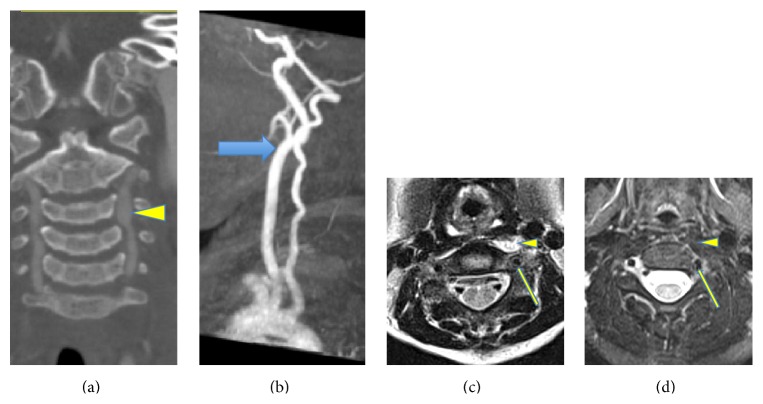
Neck imaging before and after treatment. (a) CTA coronal reformats demonstrating bilateral vertebral arteries prior to treatment. There is focal stenosis at C2-3 with poststenotic dilatation on the left side (arrowhead); the caliber of the right vertebral artery is maintained. (b) Left vertebral sagittal MRA after treatment demonstrating resolution of stenosis and poststenotic dilatation (thick blue arrow). (c) Axial MRI of cervical spine through C2 before treatment demonstrated marked adventitial thickening of bilateral vertebral arteries and focal stenosis at C2-C3 on the left side (arrow) and cervical myositis (arrowhead). (d) Posttreatment images demonstrated improvement in the adventitial thickening of bilateral vertebral arteries with improvement in the caliber of the artery at C2-3 (arrow). Note that there is complete resolution of muscular edema (arrowhead).
